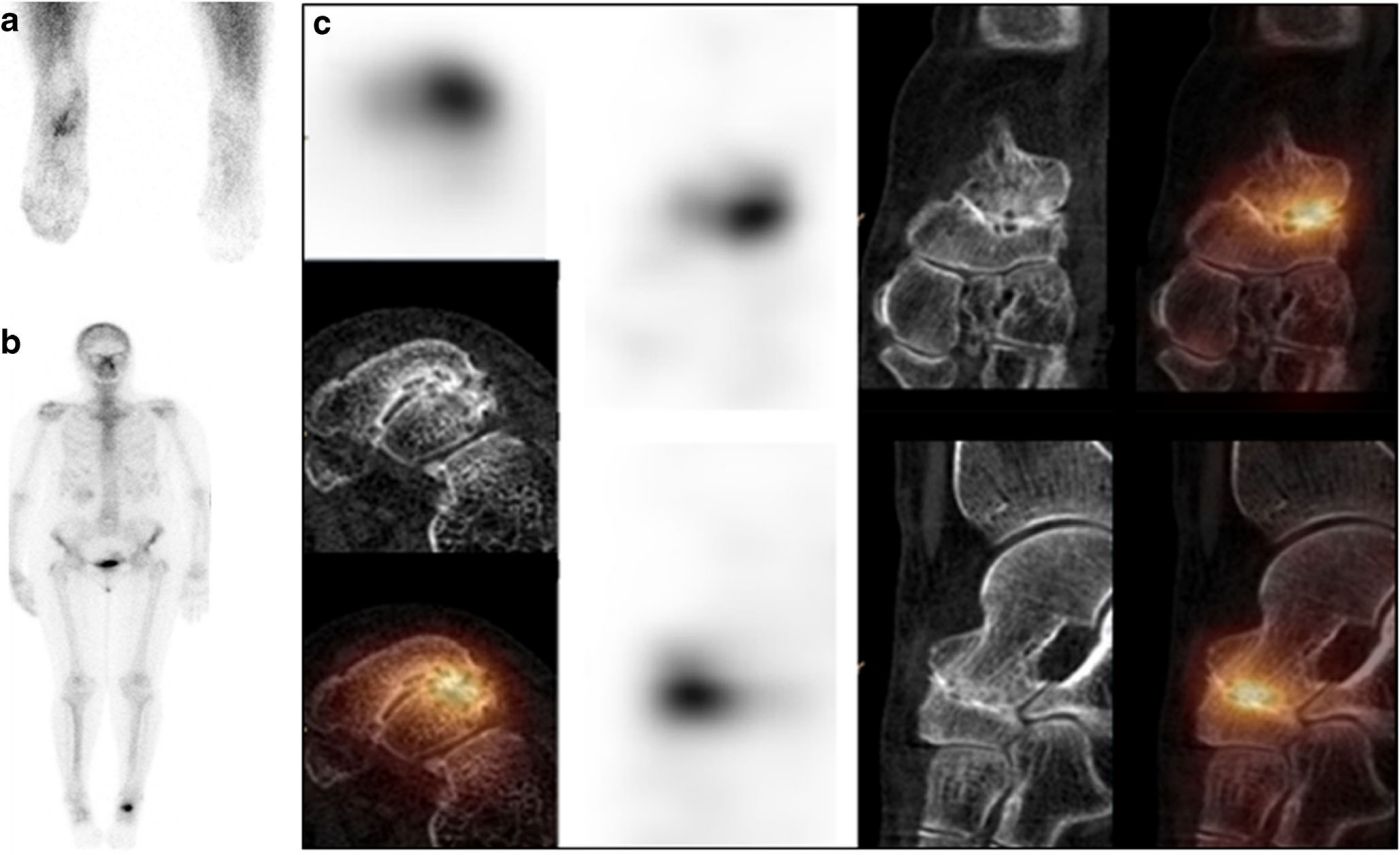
^99m^Tc-MDP bone SPECT/CT in a patient with a painful left foot. A 70-year-old patient with persistent pain in the left foot was referred for bone scintigraphy (BS) for detection of the pain generator. Early planar scan of the feet (a) and delayed whole-body scan (b) show a focus of hyperaemia and intense tracer uptake in the posterior aspect of the left tarsal region, localized by SPECT/CT (c) to severe degenerative changes in the left talonavicular joint seen on the CT component
